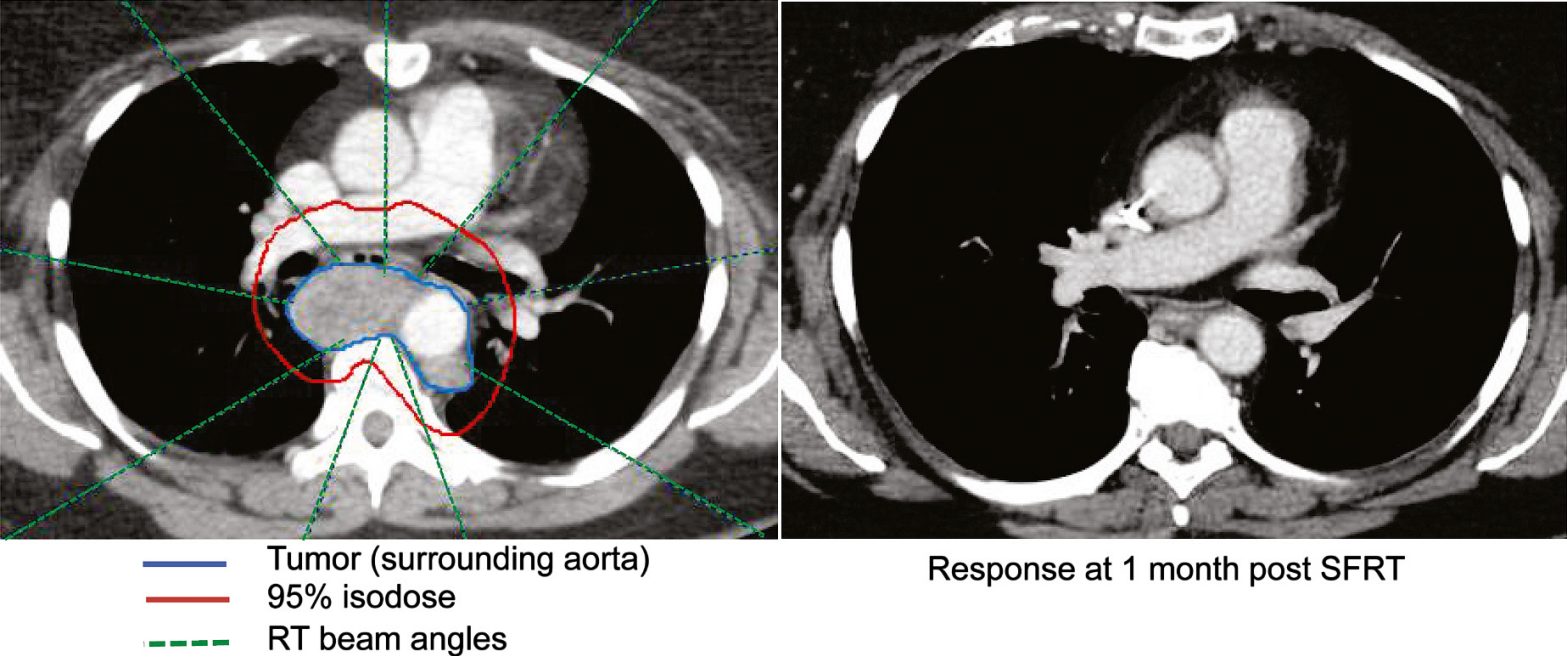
Radiotherapy plan and tumor response 1 month after SFRT. Left panel: A 56-year-old woman with recent stage IIIb MCC developed shortness of breath associated with a subcarinal paraesophageal lymph node metastasis (tumor outlined in blue, surrounding the aorta which is contrast-enhanced). She underwent SFRT, experienced no side effects from therapy, had full resolution of symptoms by day 5 after treatment, and by 1 month had a complete response as documented by CT scan (right panel). The red line represents the RT dose covering the tumor and the green dashed lines depict the nine RT beam angles directed at the tumor. The 95% isodose line in the radiotherapy plan closely conforms to the treated tumor in three dimensions, and dose was minimized to surrounding critical structures including spinal cord, heart and lungs. This tumor is included as lesion #23 in Table S1, and had not recurred as of the end of study period (11 months) or at last follow-up (22 months after SFRT). SFRT, single-fraction radiation therapy; MCC, Merkel cell carcinoma.
